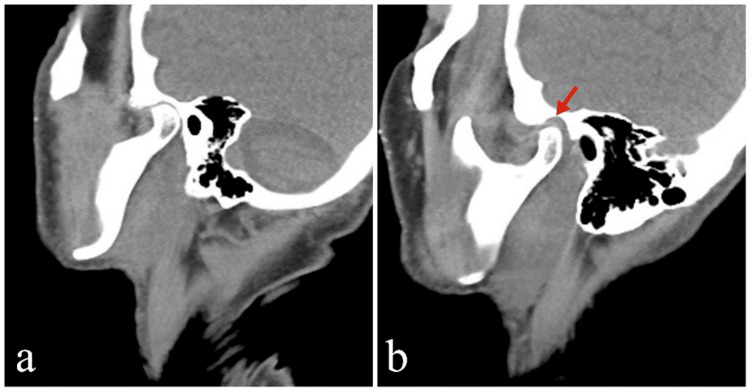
Sagittal cross-sectional images of neck computed tomography Compared to the right temporomandibular joint (a), the left temporomandibular joint (b) exhibits a widening of the joint space and an appearance compatible with inflammation in the adjacent soft tissues (red arrow). No pathology was observed in the bone tissue.
